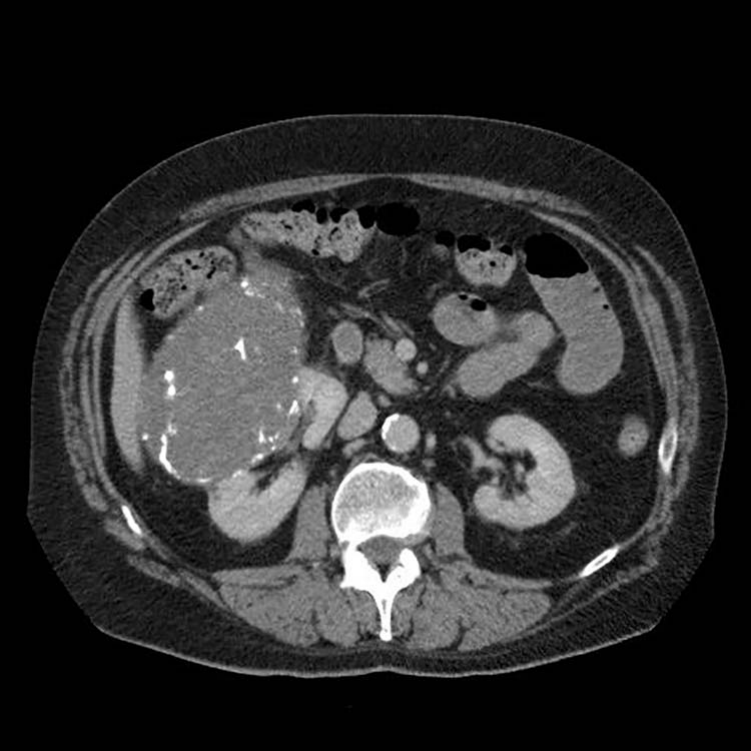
Abdominal computed tomography showing a large tumour of the right kidney and obstruction of the small intestine at the level of the proximal jejunum.
